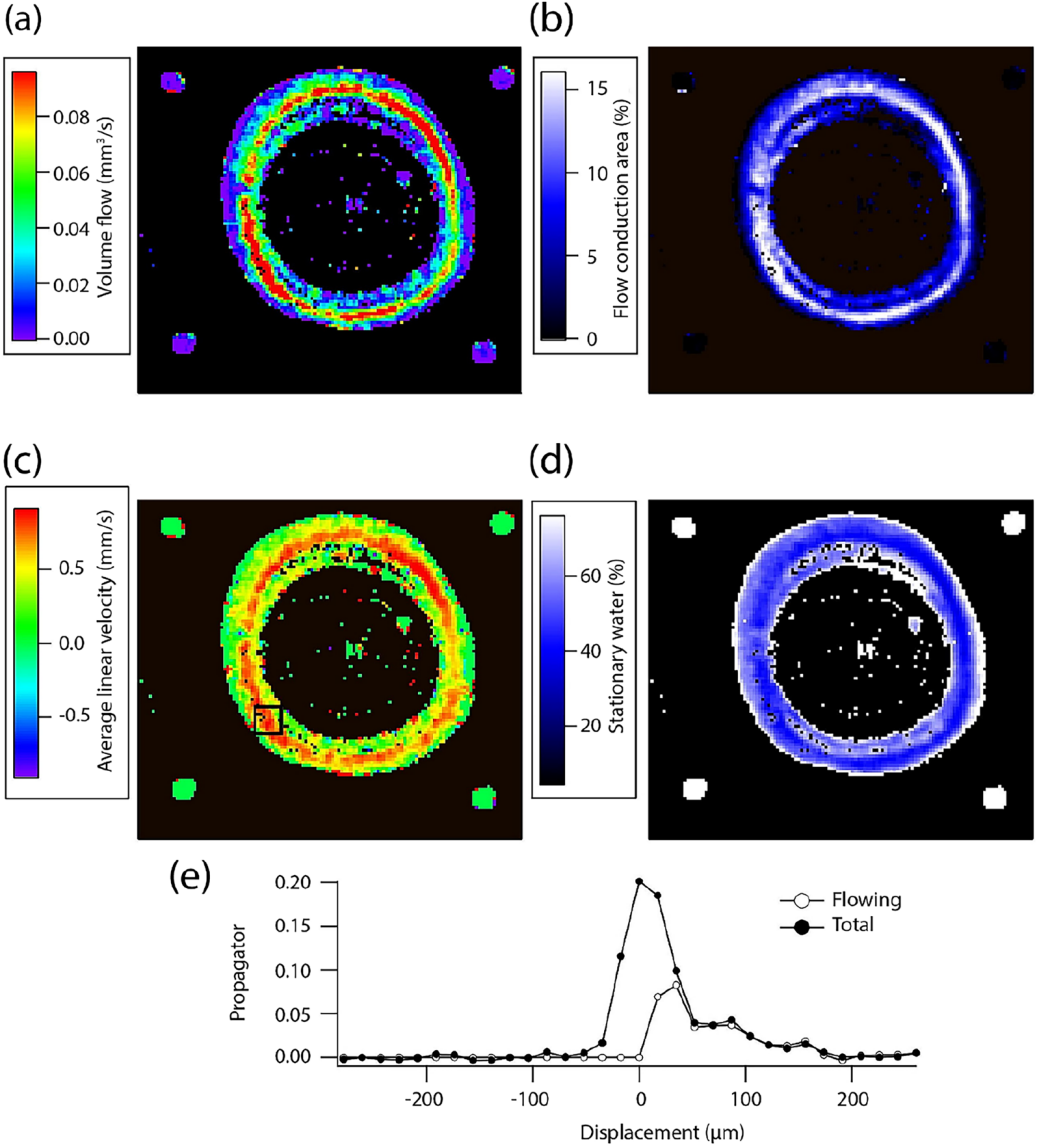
Flow parameters: volume flow (a), flow conducting area (b), average linear velocity (c), and amount of stationary water (d). The propagator displayed (e) was measured in the pixel represented by a black square in (c). These measurements were acquired with a 0.2 T-MRI on a Zelkova tree in situ (Adapted from [33] with permission from Elsevier)
